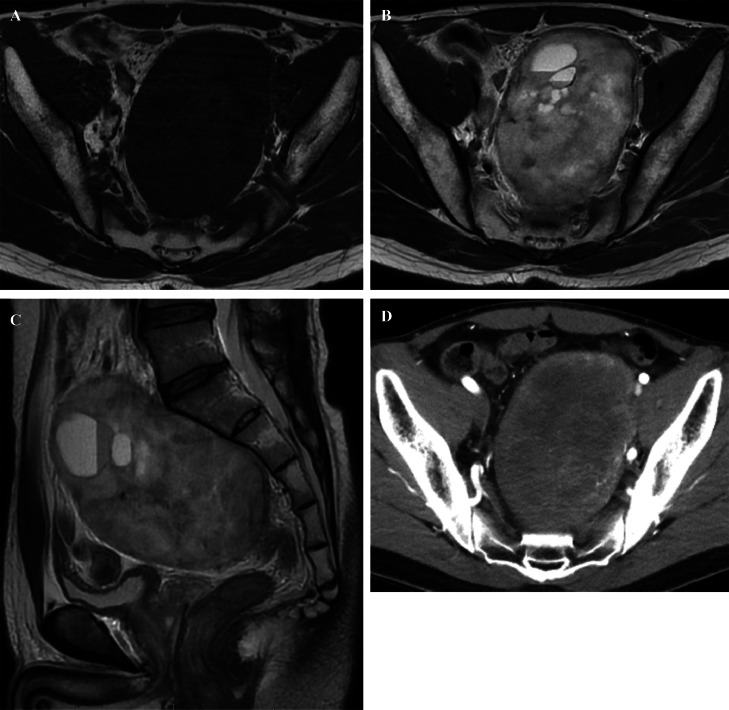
(A) MRI T1-weighted axial image showed a tumor with a well-defined marginal membrane and low signal inside the tumor. (B) MRI T2-weighted axial image showed mainly heterogeneous high signal inside the tumor, with a mixture of substantial areas and cysts. (C) MRI T2-weighted sagittal image showed the tumor in contact with the first sacrum. (D) CT showed a tumor with a diameter of 11.5 × 8 cm occupying the pelvic cavity.
